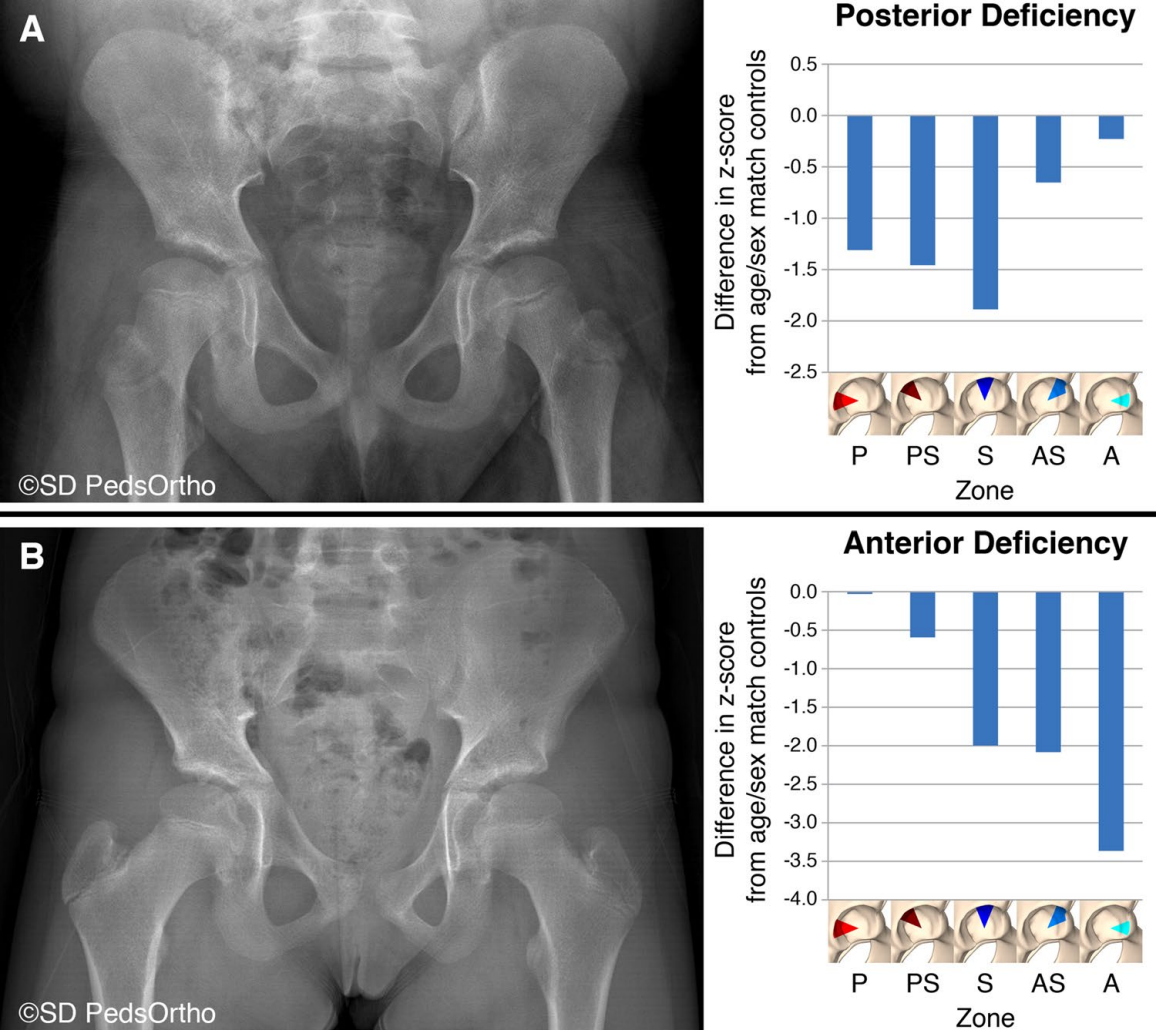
a, b Radiographs demonstrating two hips with accompanying z-scores by region of undercoverage. The hip in A demonstrates primarily posterior undercoverage in the left hip of a 16-year-old. The hip in B, on the other hand, demonstrates primarily anterior deficiency in the left hip of a 9-year-old
